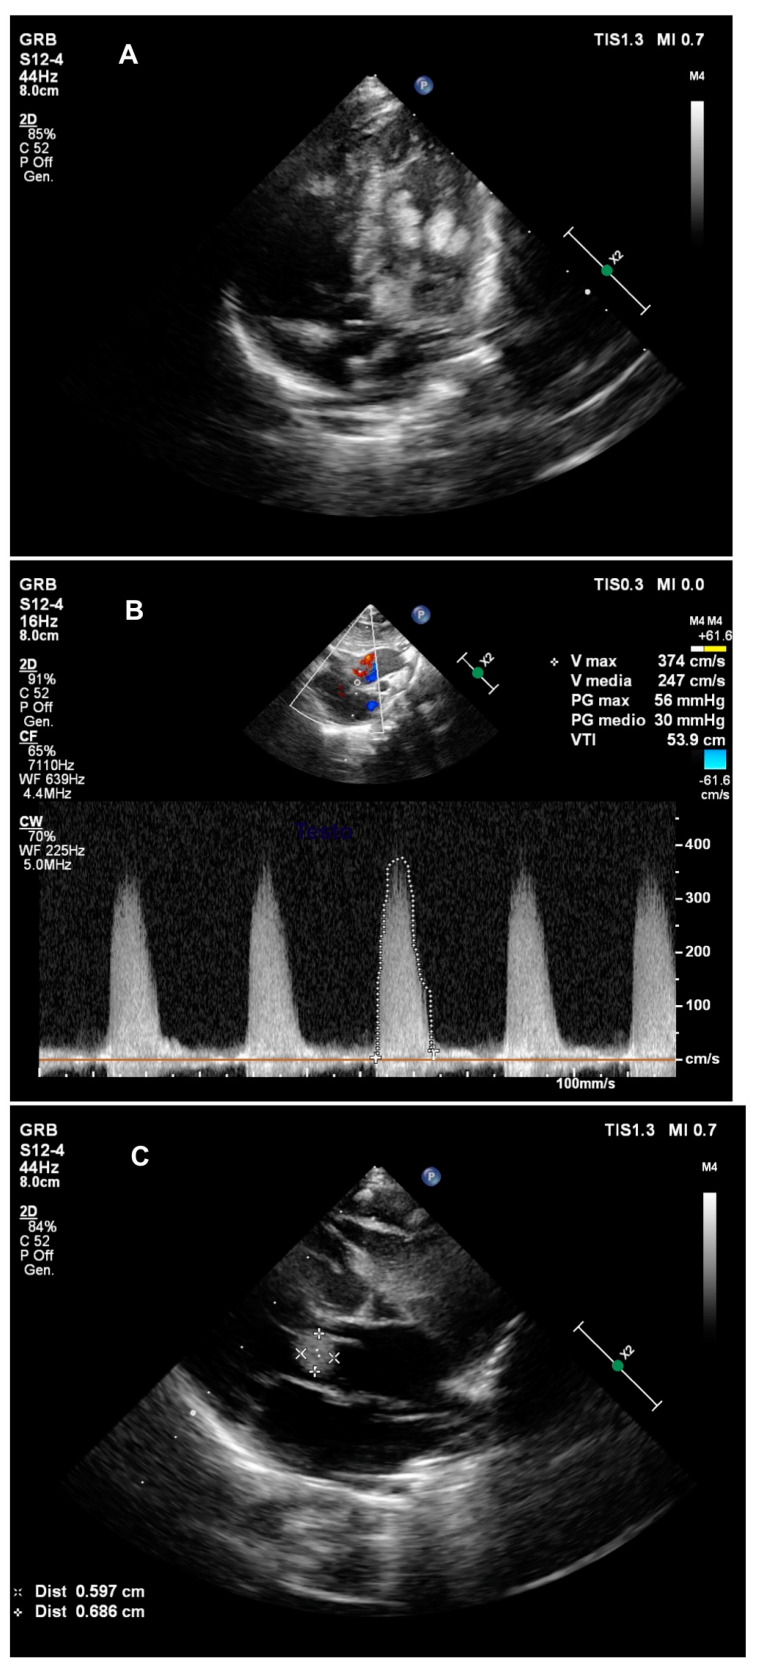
Echocardiogram on the first day of life. Panel (A): Left ventricle rhabdomyomas; Panel (B): Elevated transaortic gradient; Panel (C): Subaortic mass.
